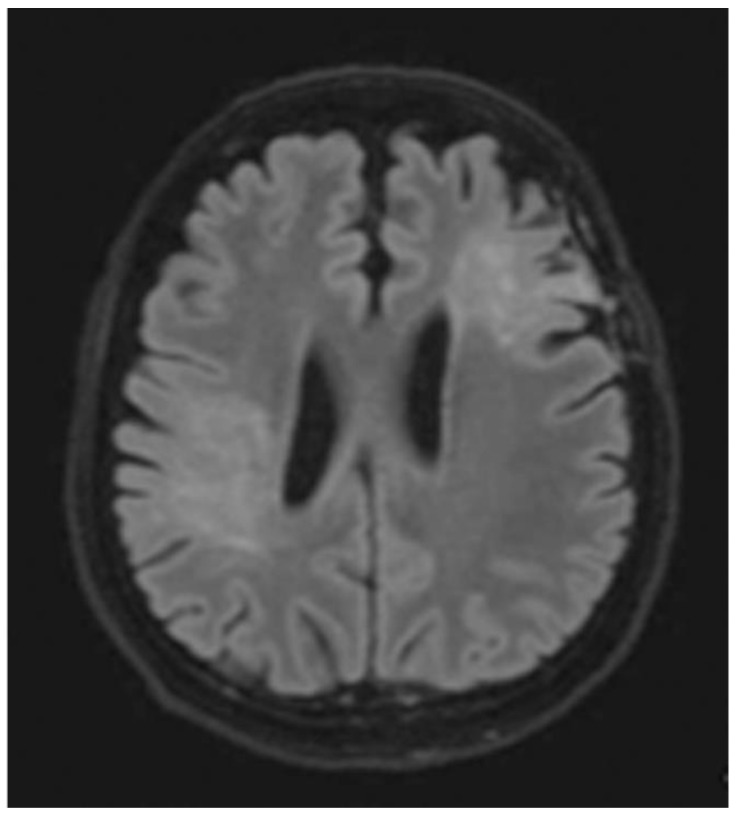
A follow-up axial post-contrast FLAIR image of the same patient performed one year after the diagnosis, showing marked improvement in lesions.
